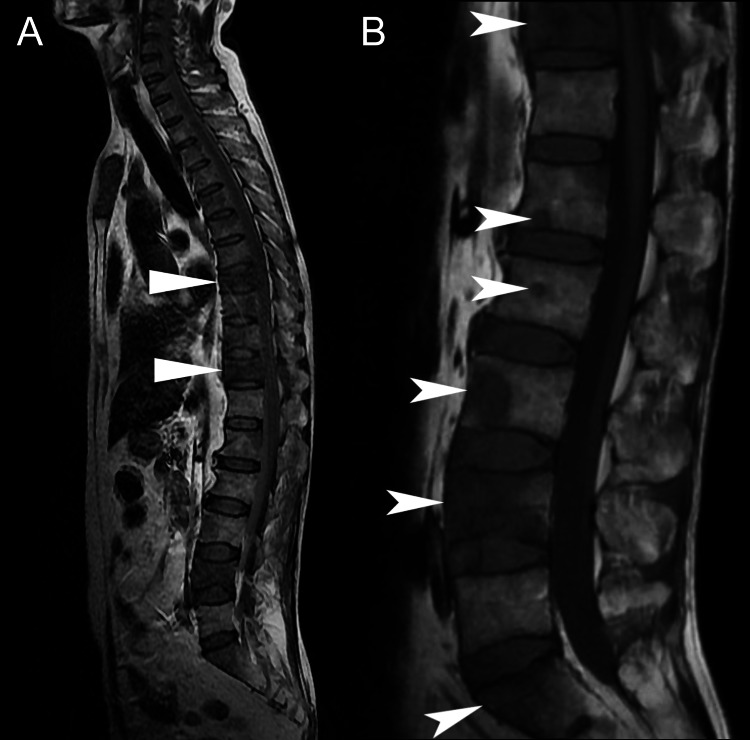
Mid-sagittal magnetic resonance imaging including a scout view (A) and T1-weighted image of the lumbar spine (B) The scout view (A) demonstrates compression fractures of T8 and T11 (triangles), with each level having 20% loss in anterior vertebral body height. In the T1-weighted view (B), heterogeneous marrow signal is evident within multiple vertebral bodies, with hypointense areas indicated in the sacrum, L4, L3, L2, L1, and T11 (arrows), suggestive of spinal metastases. Although there is mild retropulsion at T11 (not indicated), there is no significant narrowing of the central spinal canal.
